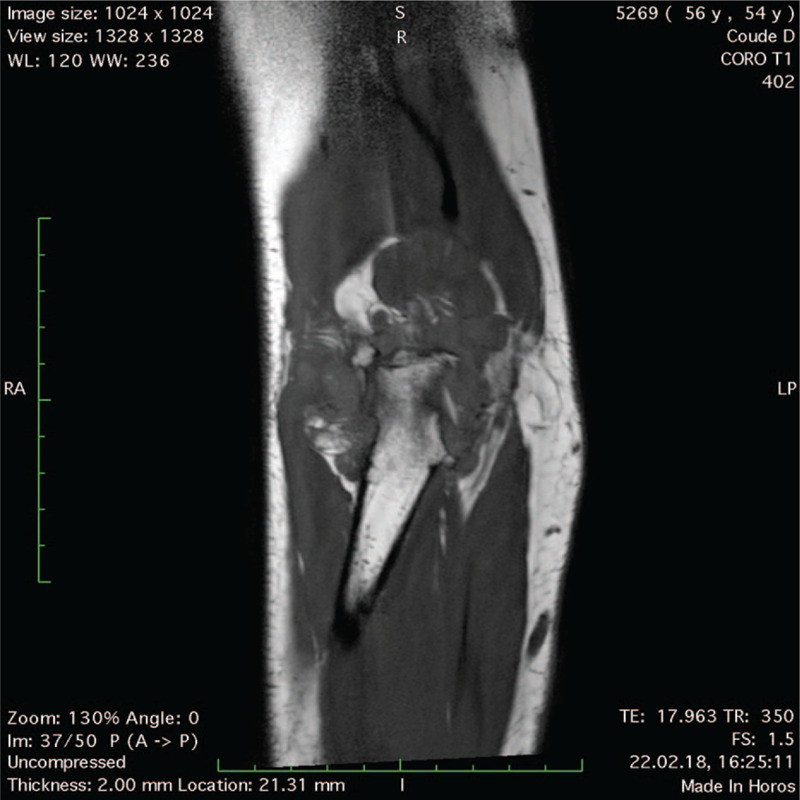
MRI T1 sequence coronal view of the right elbow showing intra-articular LA involving the whole joint.
